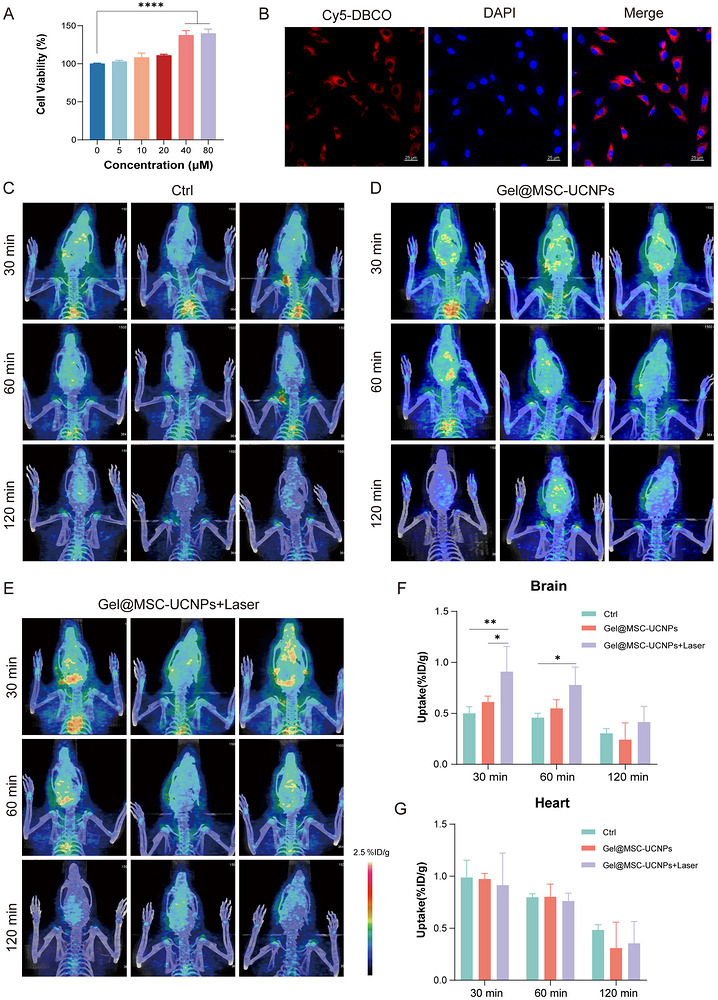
The exosome release from MSC‐UCNPs in situ. (A) Verification of the biocompatibility of Ac4ManNAz with MSCs (*n* = 3), *p* < 0.0001. (B) The covalent binding of CY5‐DBCO to nitroso‐labeled MSCs in vitro. (C–E) PET‐CT imaging enabled the detection of local exosome release in vivo. (F) Statistical analysis of ^68^Ga‐based brain tracking signals released by exosomes (*n* = 3), p* < 0.05, *p* < 0.01. (G) Statistical analysis of ^68^Ga signals in the heart/blood pool (*n* = 3).
